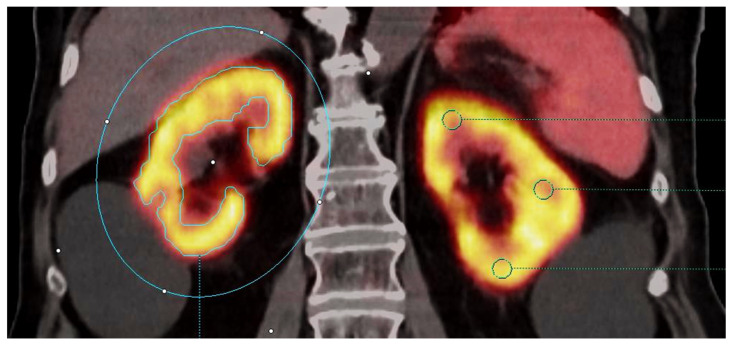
Measurement technique of renal [^68^Ga]Ga-PSMA-11 uptake in PET/CT presented in a frontal plane image. Right kidney (at the left side of the figure): Example of computer-assisted measurement showing a whole kidney VOI with isocontouring of the metabolic volume by a defined threshold (here, IC 30%) to obtain SUV and volume parameters (blue color). Left kidney: Example of manual measurements showing the three 0.5 cm diameter VOI to obtain SUV_mean_ at the predefined locations (green color). Bilateral renal cysts without PSMA ligand uptake were excluded from the measurement.
